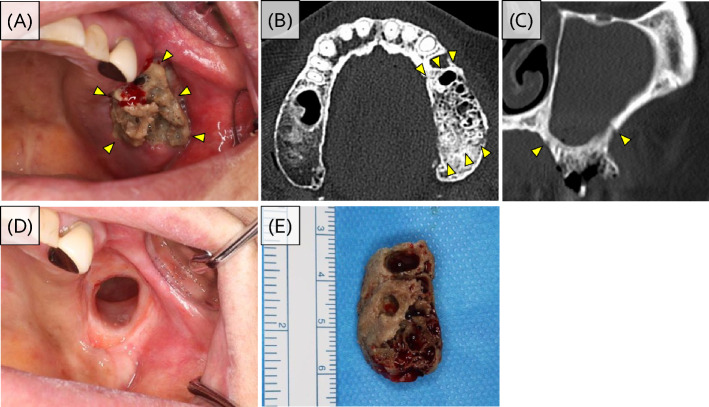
A patient with left maxillary MRONJ. A Intraoral photograph. Necrotic bone is exposed in the left maxilla (yellow arrowheads). B Axial CT image. A radiolucent thin line is visible in the osteosclerotic lesion, termed “MRONJ demarcation line” (yellow arrowheads). C Coronal CT image. MRONJ demarcation line is visible palatolingually. D Intraoral photograph taken 1 month after surgery. The wound is fully covered by the oral mucosa with the maxillary sinus opened. E Removed necrotic bone. *CT* computed tomography, *MRONJ* medication-related osteonecrosis of the jaw
